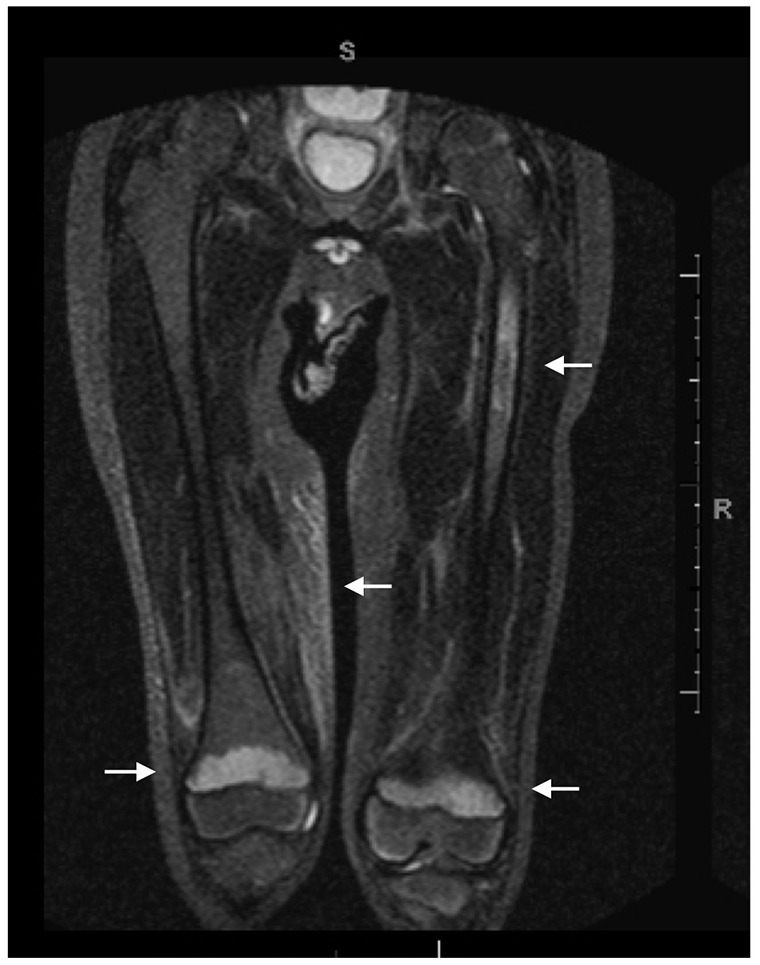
MRI STIR image of bilateral proximal lower extremities which demonstrates an abnormal
